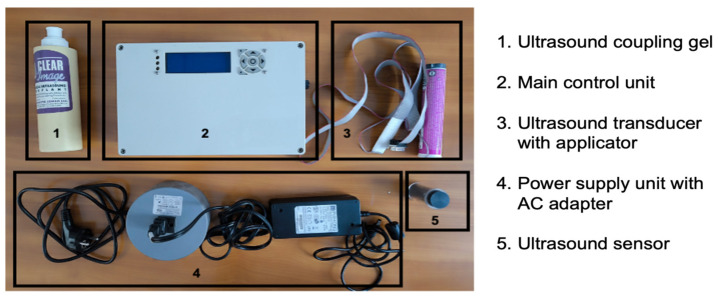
Components of the low-intensity pulsed ultrasound (LIPUS) device utilized for experimental treatment.
